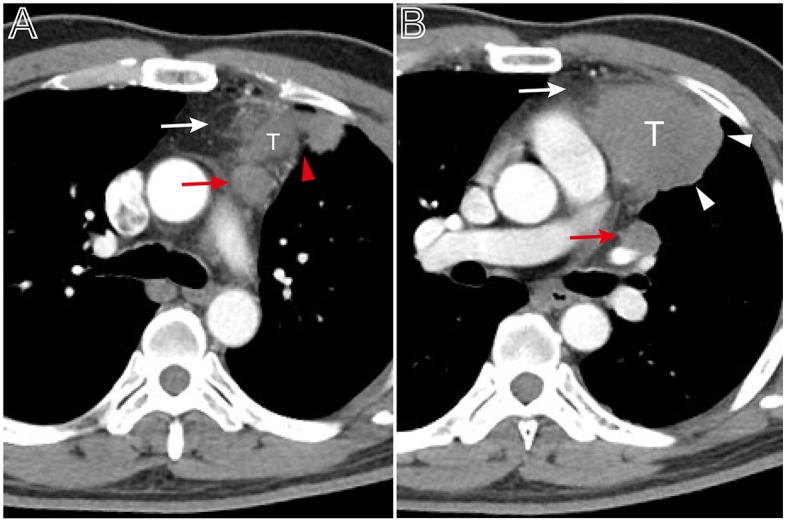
A thymoma with infiltration of surrounding fat and lymph node enlargement. Axial contrast-enhanced chest CT image obtained at the level of the pulmonary trunk demonstrated a thymoma in a 49-year-old man (Masaoka–Koga stage IVb and WHO classification B3). (A,B) Showed the thickened pleura (red arrow head), infiltration of surrounding fat (white arrows), and bulging margin (white arrow head). The lymph node enlargement appeared at the superior level of the lesion (A, red arrow) and at the level of left hilum (B, red arrow).
